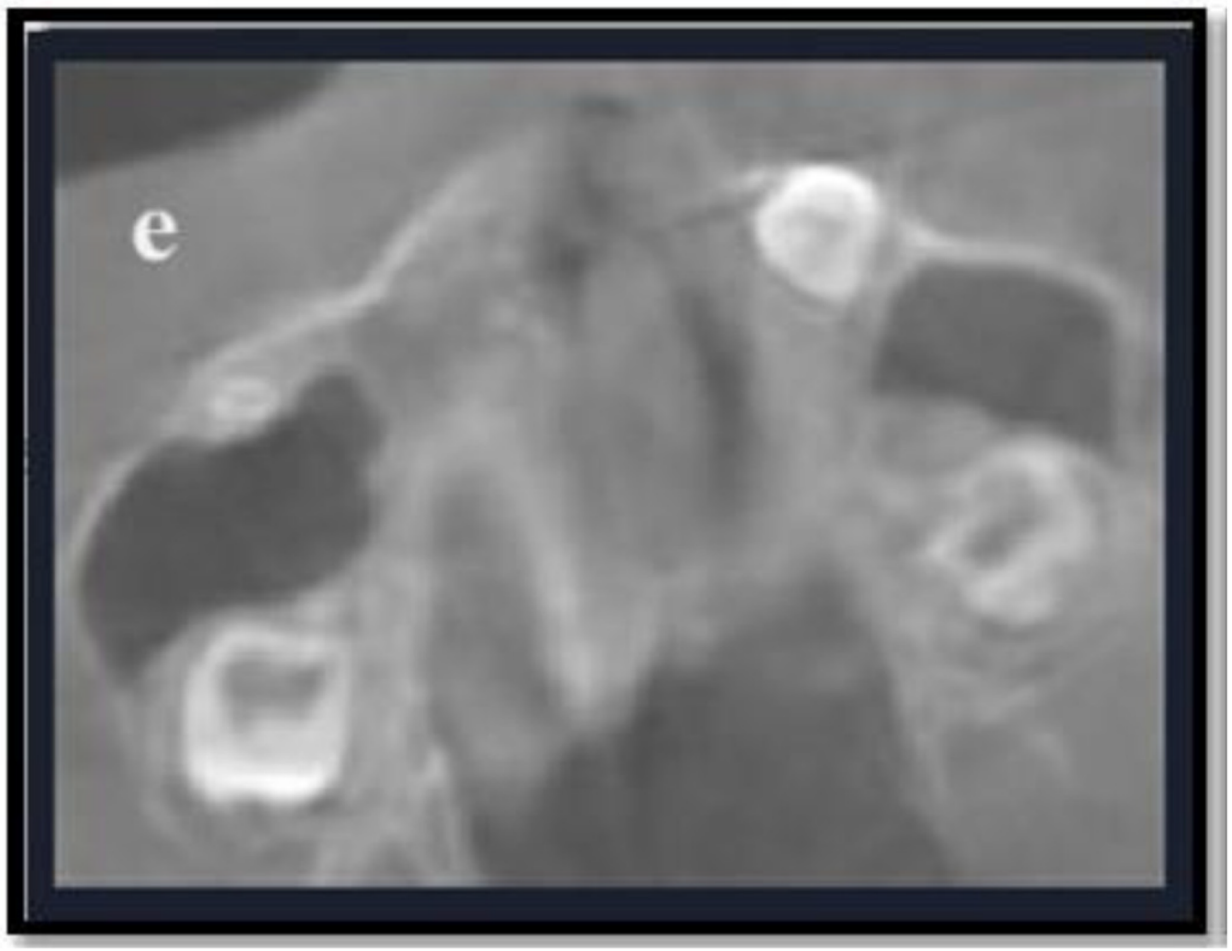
Represents the following stage of MPS in axial view of CBCT images: (e) Complete fusion of MPS is seen within the intermaxillary region.
